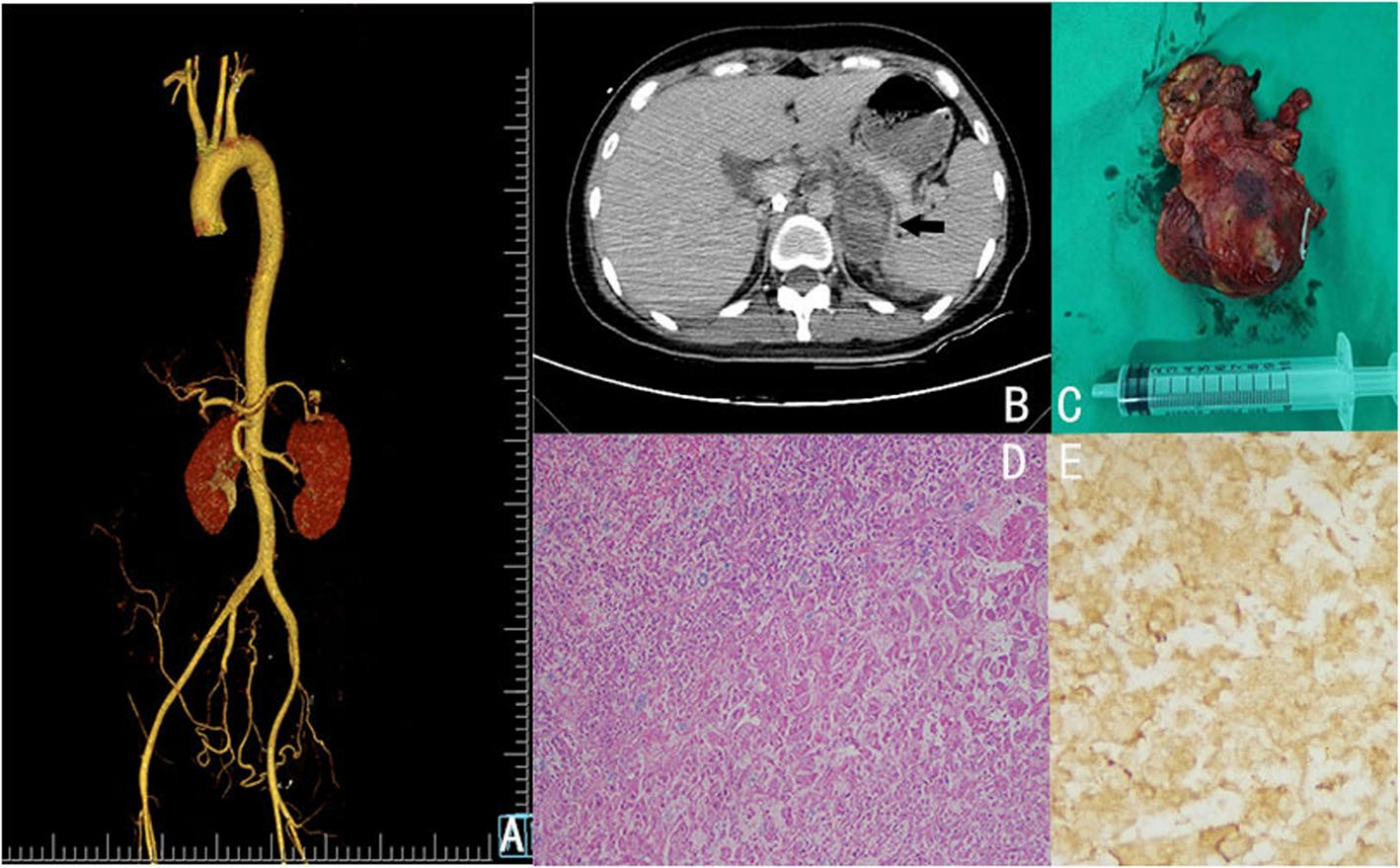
Computed tomography angiography of the thoracoabdominal aorta (A), a whole-abdominal enhanced CT scan revealing a cystic mass in the left adrenal region with hemorrhage (B, indicated by an arrow), a left-sided adrenal pheochromocytoma after resection (C), and pathological results (D, E)
